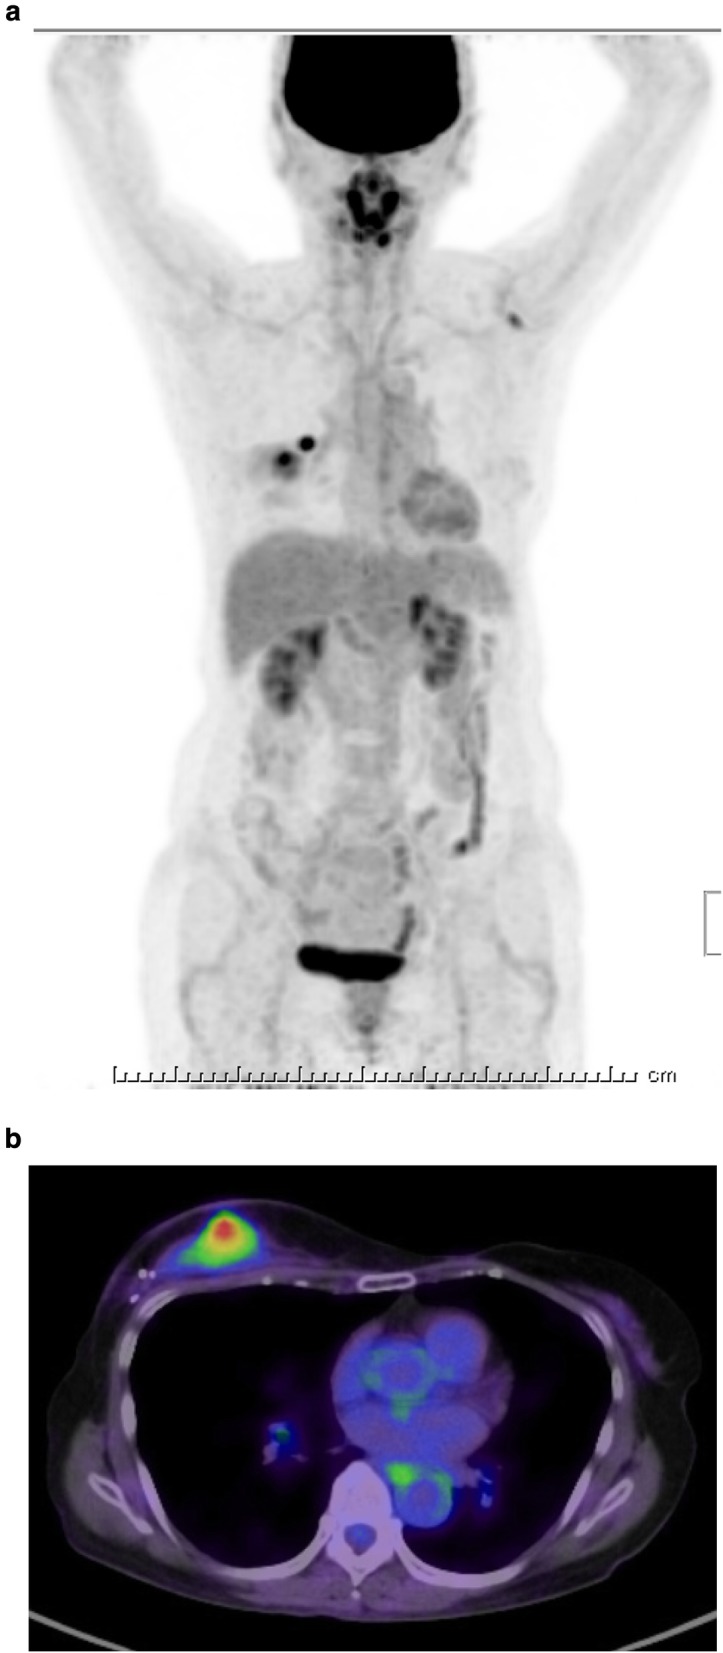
a PET/CT before the surgery showed no metastatic disease. b PET/CT showed accumulation in the tumor
